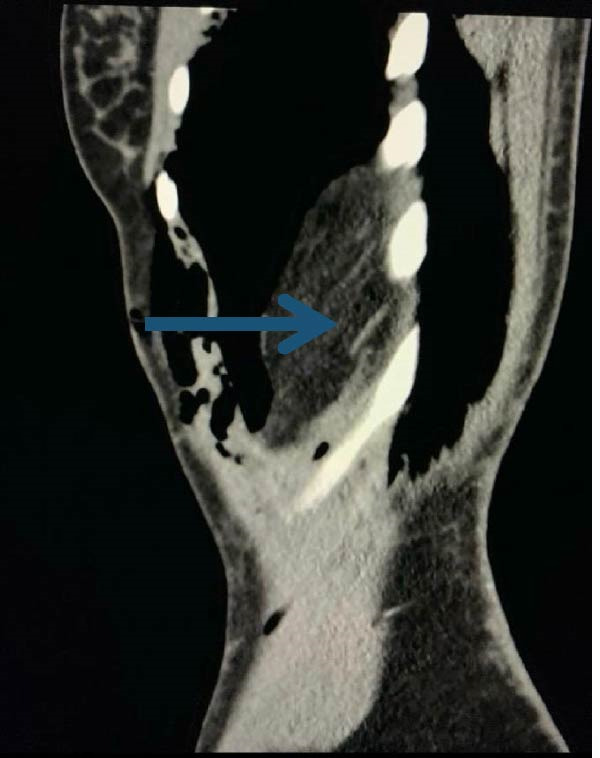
Sagittal computed tomography of the abdomen. The blue arrow shows hemidiaphragmatic disruption with herniation of abdominal viscera into the left hemithorax. *Source:* Image obtained during the study.
